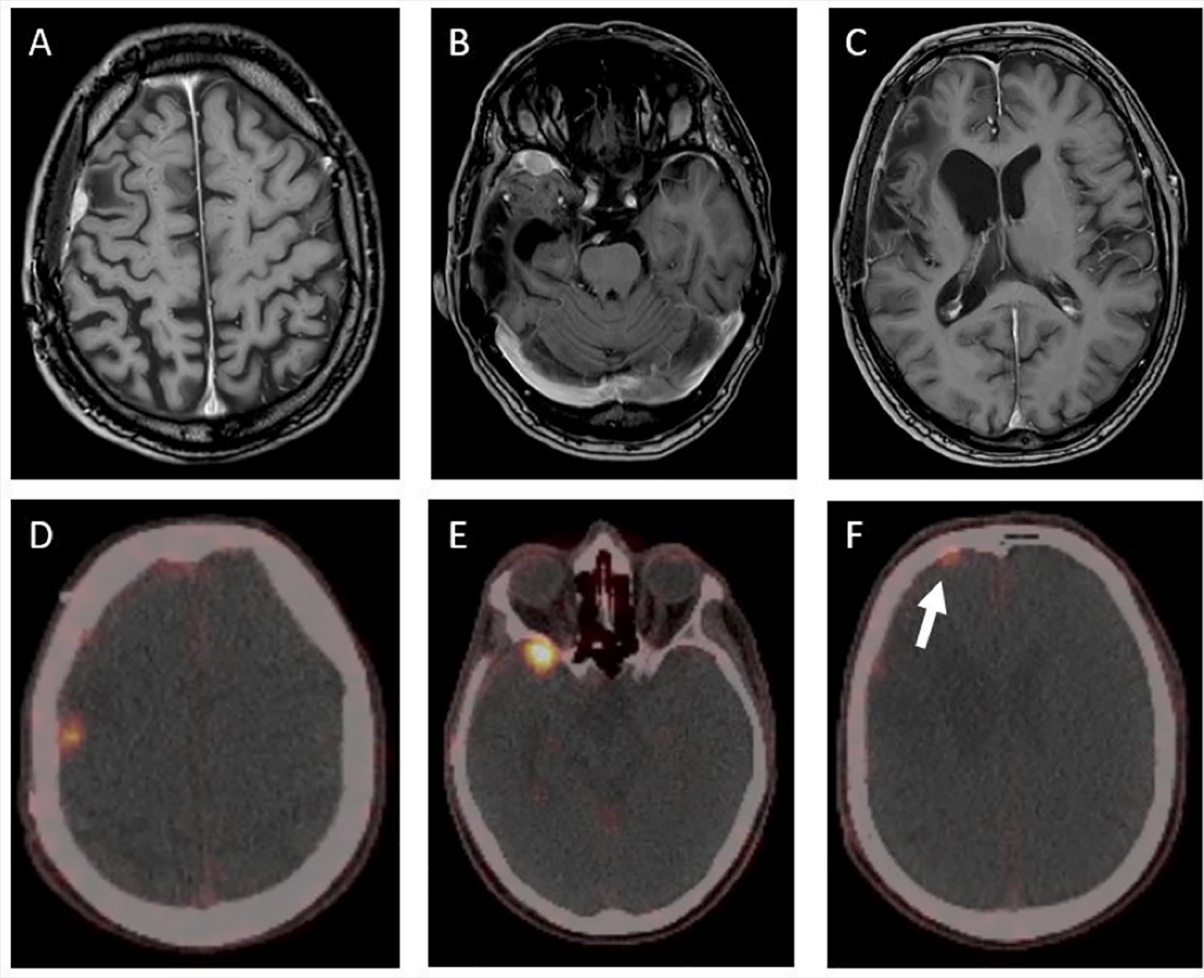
T1-contrast-enhanced MRI and ^68^Ga-DOTATATE-PET/CT showing three PET-positive lesions in different locations (D–F). The contrast- enhanced MRI (A–C) showed no clear correlate for the displayed PET-positive right frontopolar lesion (white arrow). The maximum standardized uptake value (SUV_max_) was lower in the previously irradiated lesion (D) compared to those of the untreated lesions (E, F) (SUV_max_ of 1.9 *vs*. SUV_max_ of 9.8 and 3.0).
